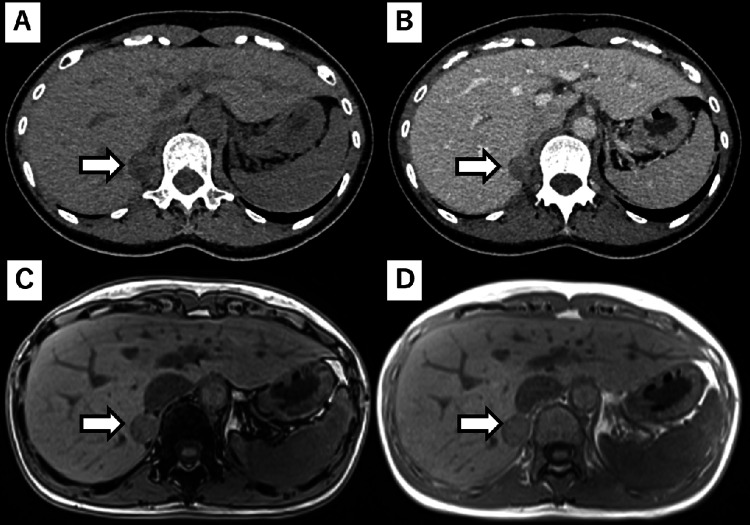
Adrenal gland tumor images at the PA diagnosis Right adrenal tumor images at the time of PA diagnosis were obtained using unenhanced CT (A), enhanced CT (B), out-of-phase MRI (C), and in-phase MRI (D). PA: Primary aldosteronism.
